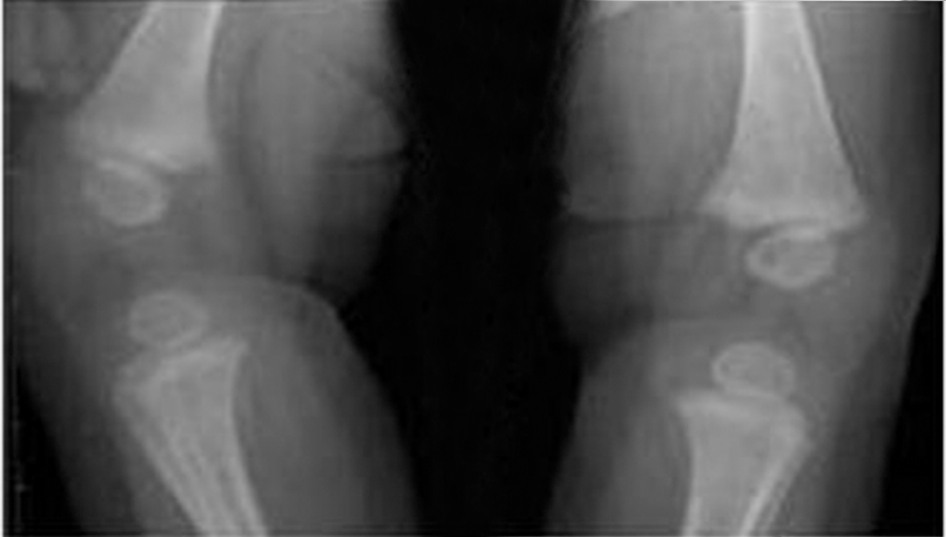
X-ray control at 4 months of age: resolution area of osteolysis
